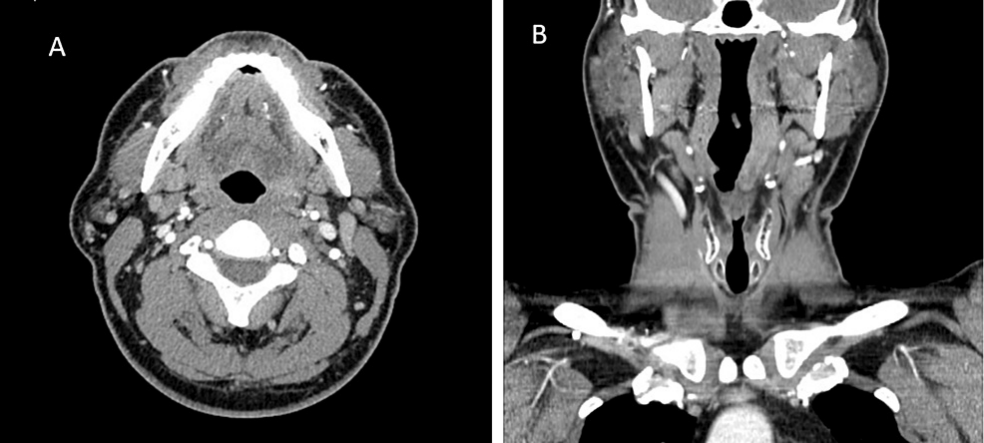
One month post incision and drainage, CT head and neck shows resolution of the previously observed retropharyngeal abscess with minimal residual changes of the oropharynx
